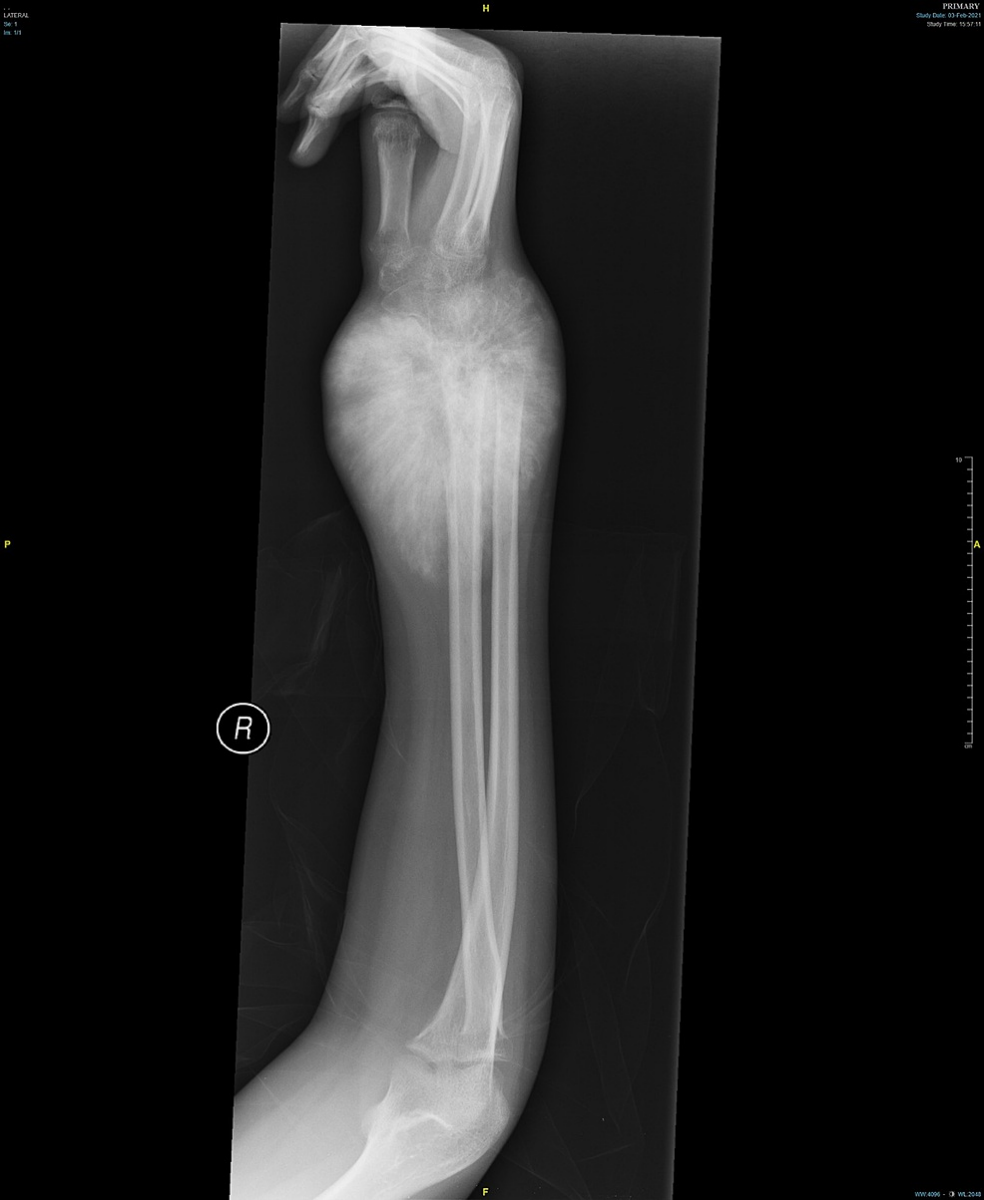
X-ray of the right upper limb shows moth-eaten appearance of distal radius metaphyseal region, lytic lesion, and cortex breakage at the radius styloid.
